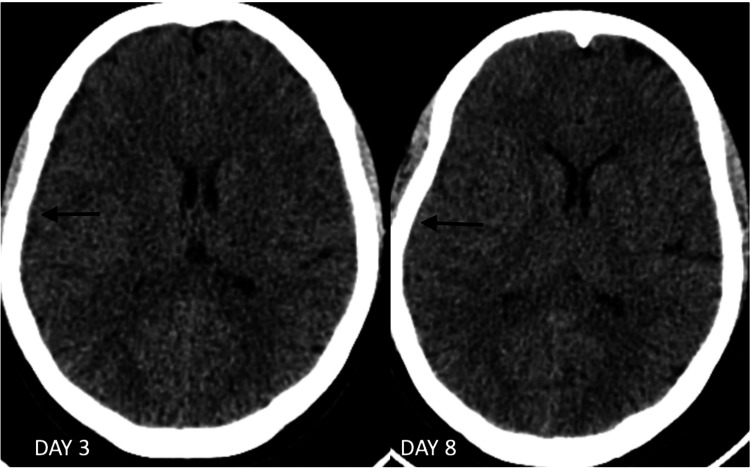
Computed tomography (CT) brain plains: on days three and eight of treatment.
